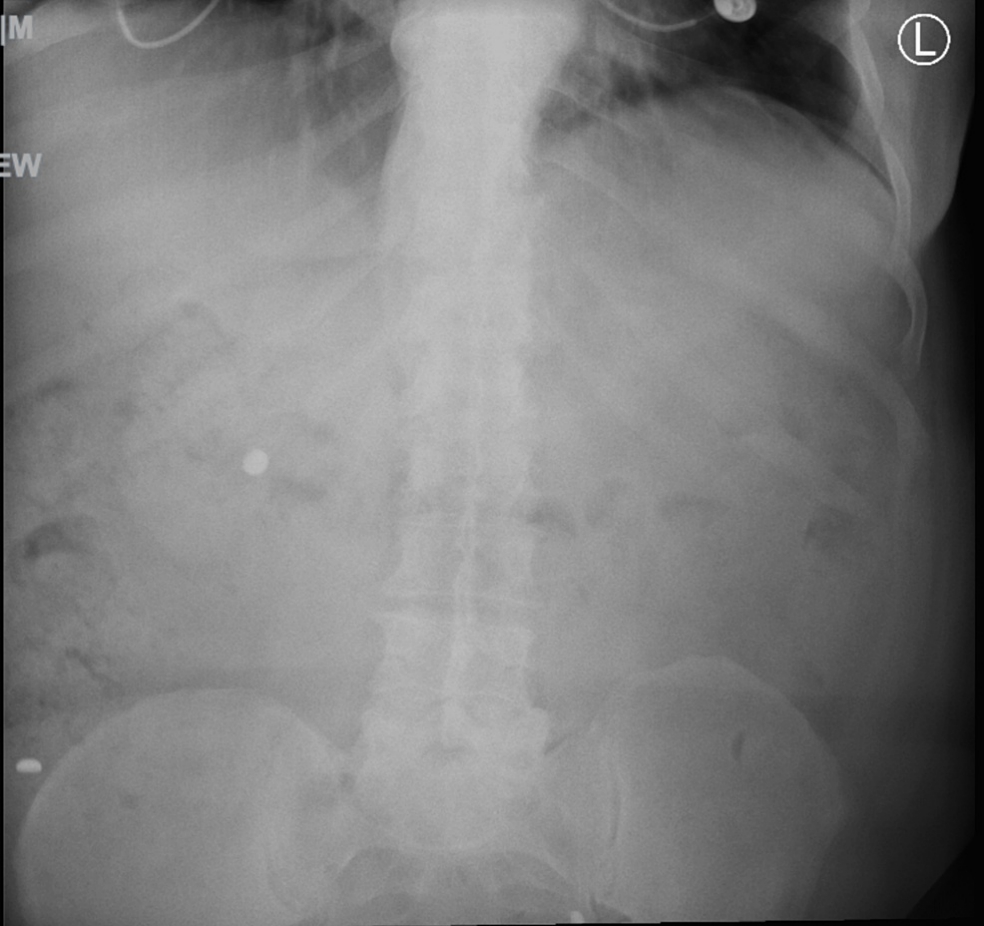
More hyperdense round objects seen on the radiograph one day after the colonoscopy
